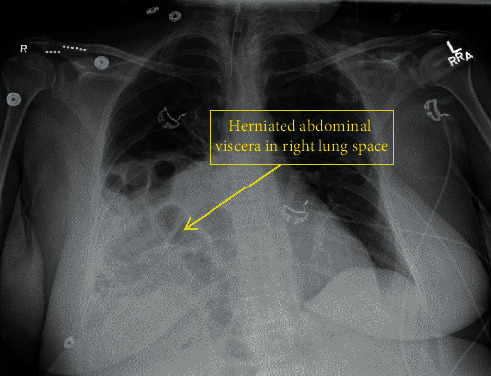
Chest X-ray revealing large right Morgagni hernia containing fat and several loops of small bowel. Associated right lung volume loss and atelectasis.
